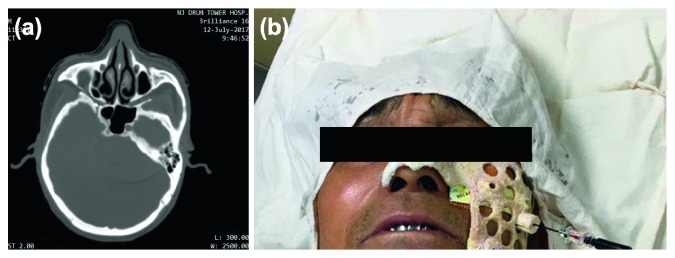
Clinical application of the template. After the process of skin preparation and draping, the template was placed on the patient's face stably and local anesthesia was given through guide cannula. Then, the needle was punctured to the measured depth through guide cannula. A CT scan was conducted to confirm the correct cannulation before the routine treatment process.
